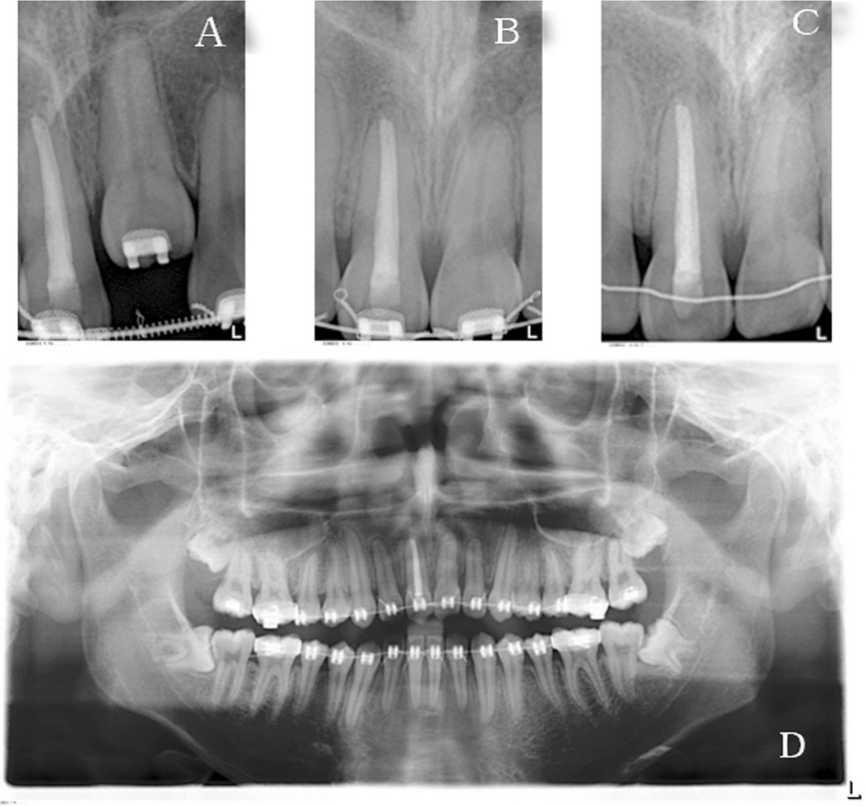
Radiographic images of the tooth at the various treatment stages. (A) Periapical radiograph showing the condition of the left central incisor was suitable for subluxation; (B–C) No resorption evident in the roots of the central incisors; (D) Panoramic radiograph taken to check the condition of the anterior teeth.
